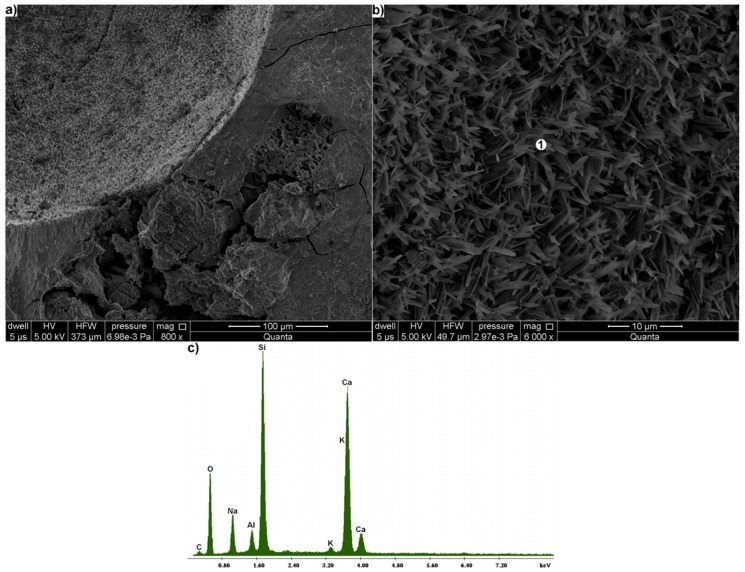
Microstructure of mortar: (a) pore in mortar at 800× magnification; (b) products of alkali-silica reaction, filling it; (c) X-ray microanalysis in point 1.
